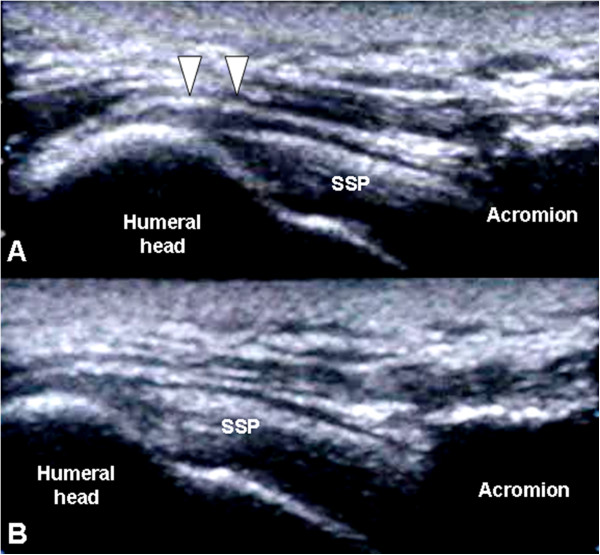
Subdeltoid fluid and impingement before and after implants removal. A 31-year-old man who received hook plate fixation of a right distal clavicle fracture developed shoulder impingement syndrome. (A) Musculoskeletal sonography revealed subacromial/subdeltoid (SASD) bursitis with flowing fluid before implant removal (arrowhead). (B) Four weeks after implant removal, the same patient was reevaluated. Sonographic finding showed unobstructed passage of the humeral head into the acromion and disappearance of the flowing fluid. SSP, supraspinatus tendon.
